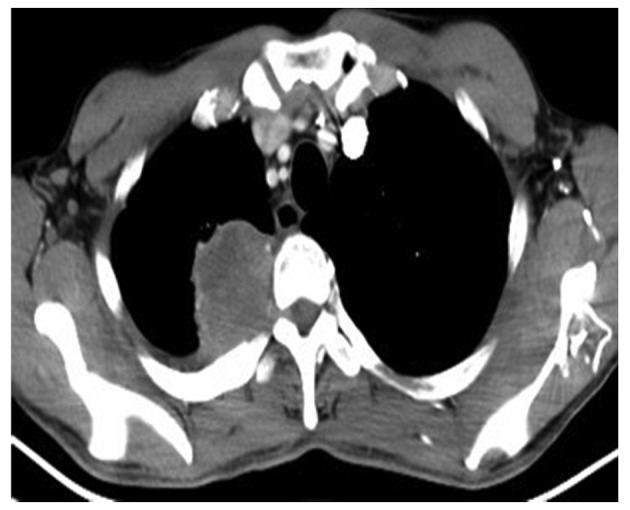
Computed tomography of the chest showed a large mass in the posterior mediastinum.
